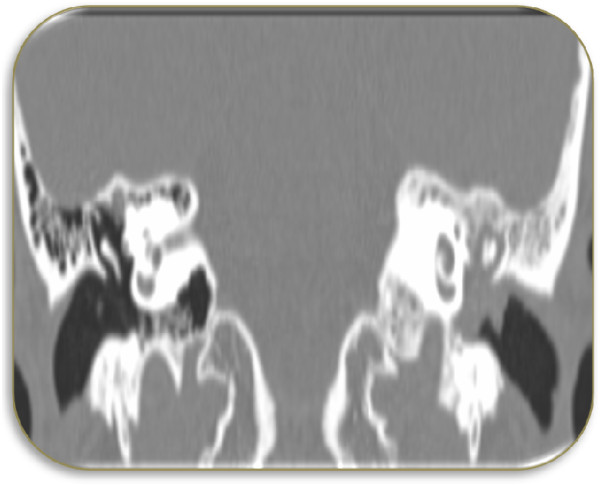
Computed tomography scan of inner part of left external auditory canal. An irregular soft tissue lesion seen in the inner part of the left external auditory canal just lateral to the tympanic membrane, soft tissue density is also seen implicating the Prussak’s space, epitympanum, mesotympanum and hypotympanum.
